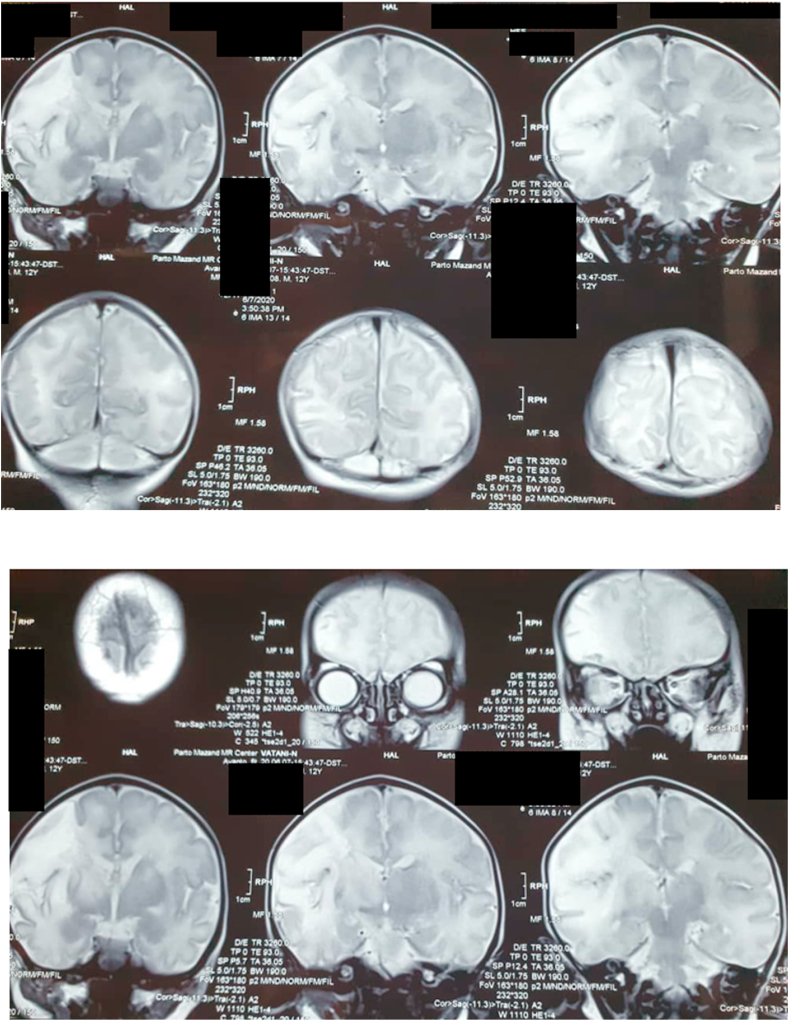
MRI results. Temporoparietal lesion (due to asphyxia) in imaging.
